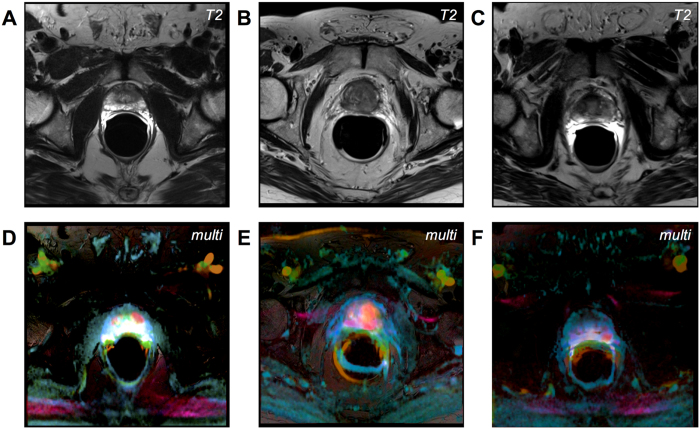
More examples of prostate cancer patients. (A–C) T2-weighted images. (D–F) corresponding multivariate visualization. Prostate tumors take on a red hue.
